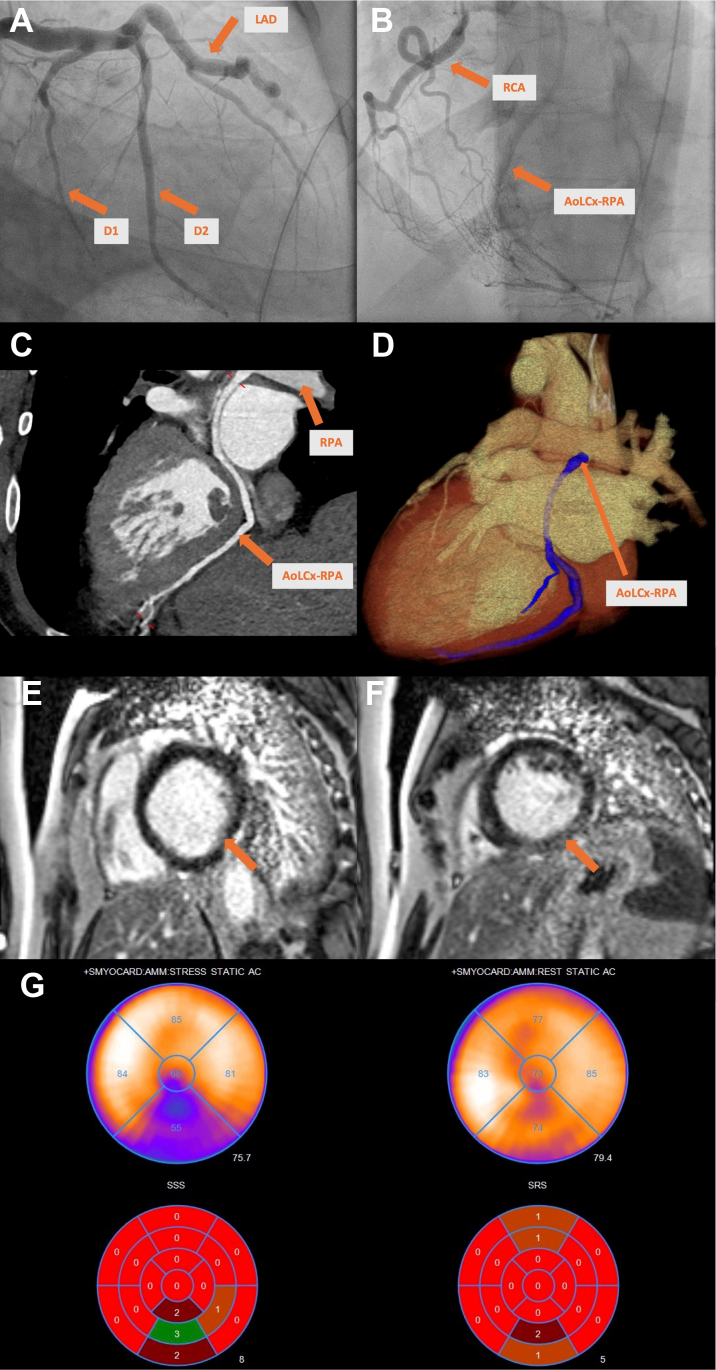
Anomalous Left Circumflex Artery From Right Pulmonary Artery: Multimodal Imaging Overview (A) Coronary angiogram (RAO caudal): dilated LAD with absent LCx. (B) Coronary angiogram (LAO cranial): RCA collaterals and delayed LCx filling. (C and D) Cardiac CT: LCx originating from RPA (2.3 cm from bifurcation) with fistulous connections to LAD and RCA. (E) cMRI: subendocardial delayed enhancement in basilar inferior wall. (F) cMRI: delayed enhancement, mid-apical lateral, inferior LV walls. (G) PET-CT (stress, rest): severe reversible ischemia (18% myocardium) from basal-apical, inferior wall with mild hypokinesis inferior segments during stress. EF: prestress 55%, poststress 54%, TID ratio 1.06. Lowest perfusion in RCA territory.
